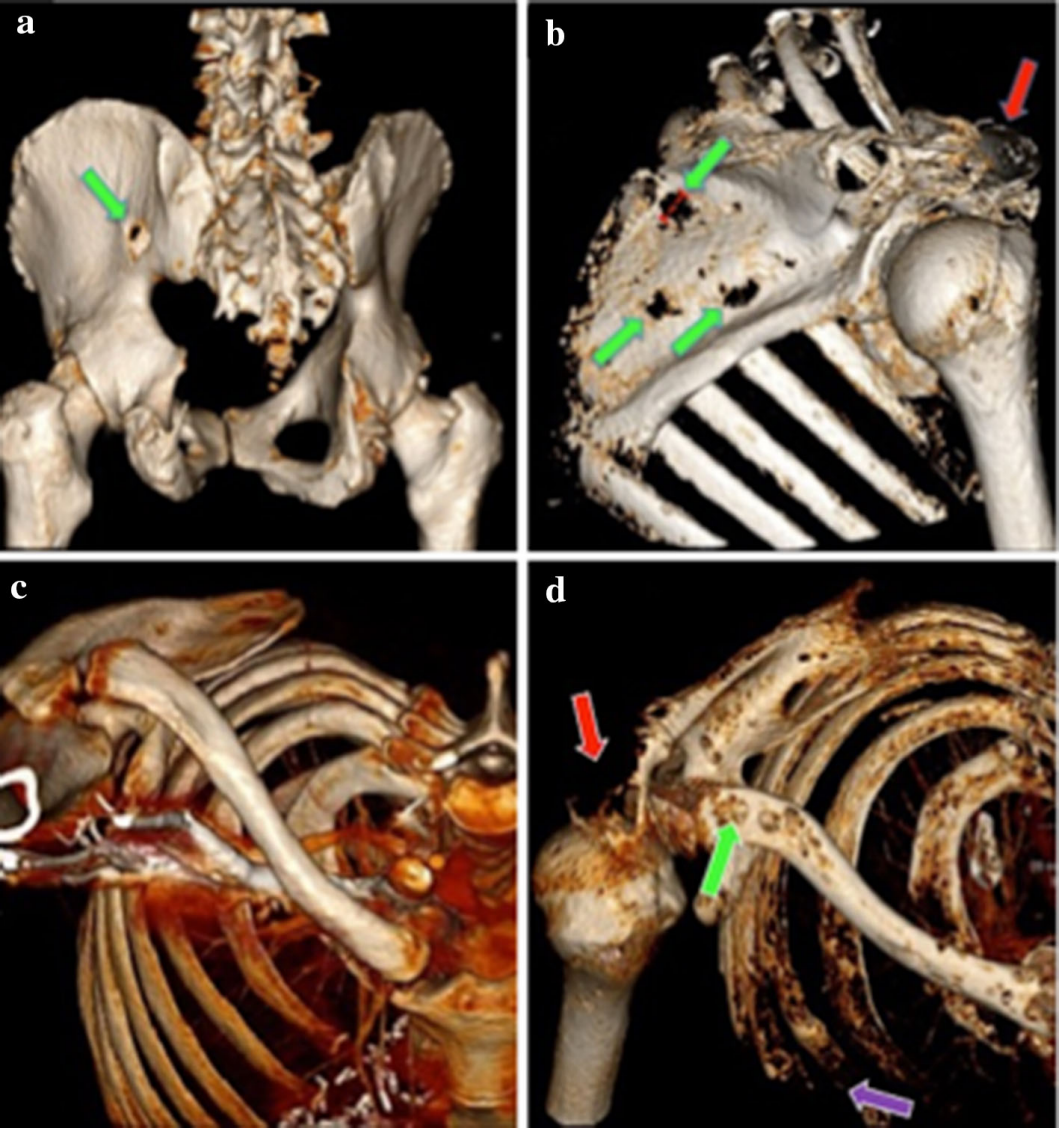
3D reconstructions of computerised tomography (CT) images using standard diagnostic settings demonstrating two patients with widespread myeloma-induced bone disease, leading to potential serious consequences. a Lytic lesion penetrating through the ischium (green arrow). b Multiple lytic lesions throughout the scapula (green arrows) with the acromion completely destroyed by myeloma bone disease (red arrow). c Example of normal bone from the shoulder, clavicle and ribs. d Contrast image of the patient riddled with lytic lesions due to myeloma bone disease. The acromion process is destroyed (red arrow), multiple lytic lesions are present throughout the clavicle (green arrow) and the anterior ribs have been destroyed (purple arrow) (Color figure online)
